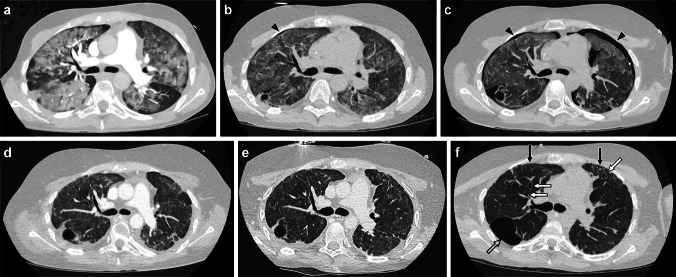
A 19-year-old male with history of acute myeloid leukemia and multiple hematopoietic stem cell transplants, most recently 18 months prior, who received cefepime and vancomycin for leukocytosis identified during work-up of poor weight gain, and who upon completion of his cefepime course developed acute respiratory failure. a Axial CT image with contrast showing extensive bilateral consolidation and ground-glass opacity typical of diffuse alveolar damage. b-f Axial CT images from five chest CT exams obtained during the subsequent year showing evolution of diffuse alveolar damage with initial replacement of consolidation by ground-glass opacity and reticulation, subsequent resolution of ground-glass opacity and decrease in reticulation, and ultimately stable reticulation (*black arrows*) and bronchiectasis/bronchiolectasis (*white arrows*) compatible with fibrosis. Pneumothoraces were also transiently seen (*arrowheads*) and a right lower lobe pneumatocele developed (*open arrow*) during the clinical course. After extensive work-up, a diagnosis of medication-induced lung disease was thought most likely and steroids, abatacept, and infliximab were administered during the clinical course with clinical improvement noted
